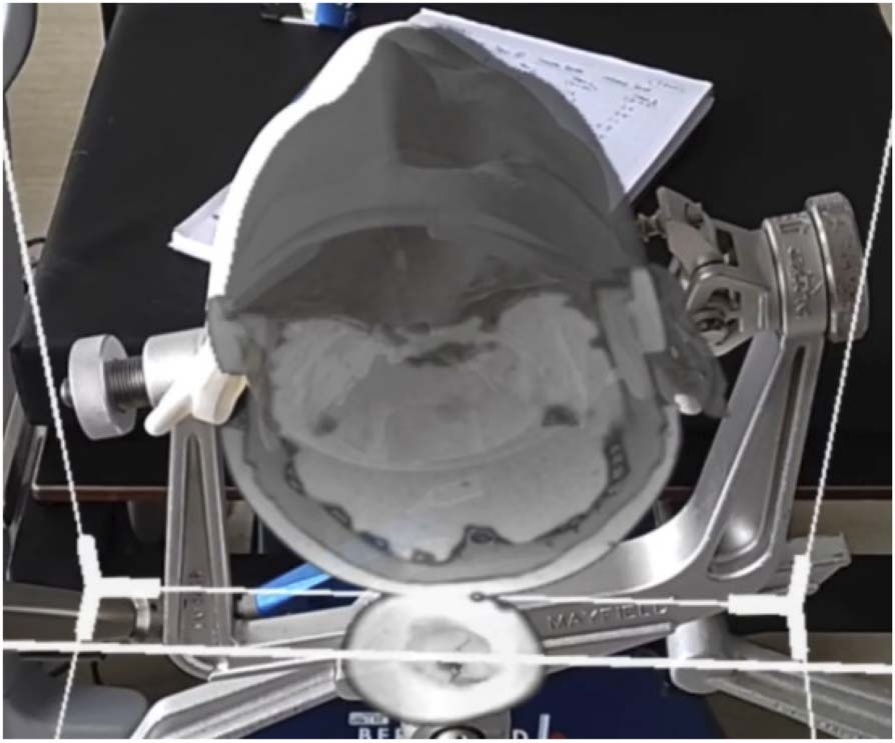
Superimposed MRI image being placed on the Realistic Operative Workstation for Educating Neurosurgical Apprentices model.
